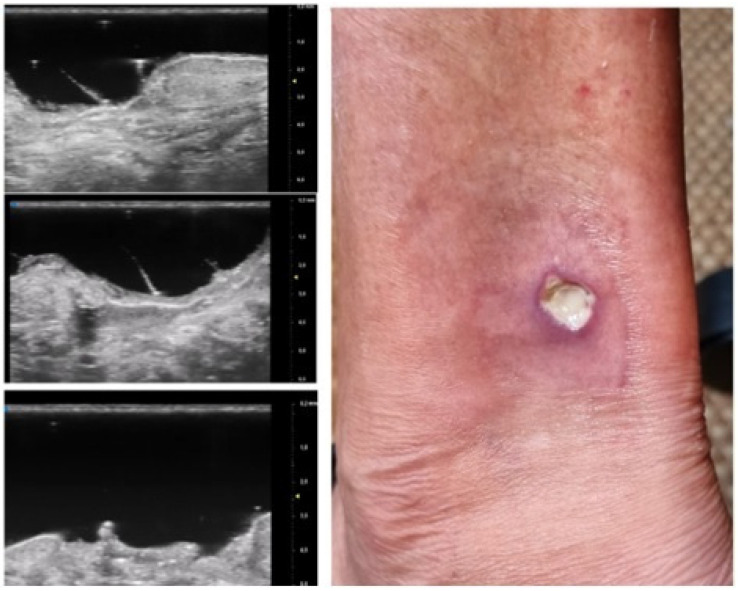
Patient affected by ulcerative Pyoderma Gangrenosum (PG) of the calf during the non-inflammatory phase: clinical aspect of the wound and its Ultra-High Frequency UltraSound (UHFUS) correlates.
